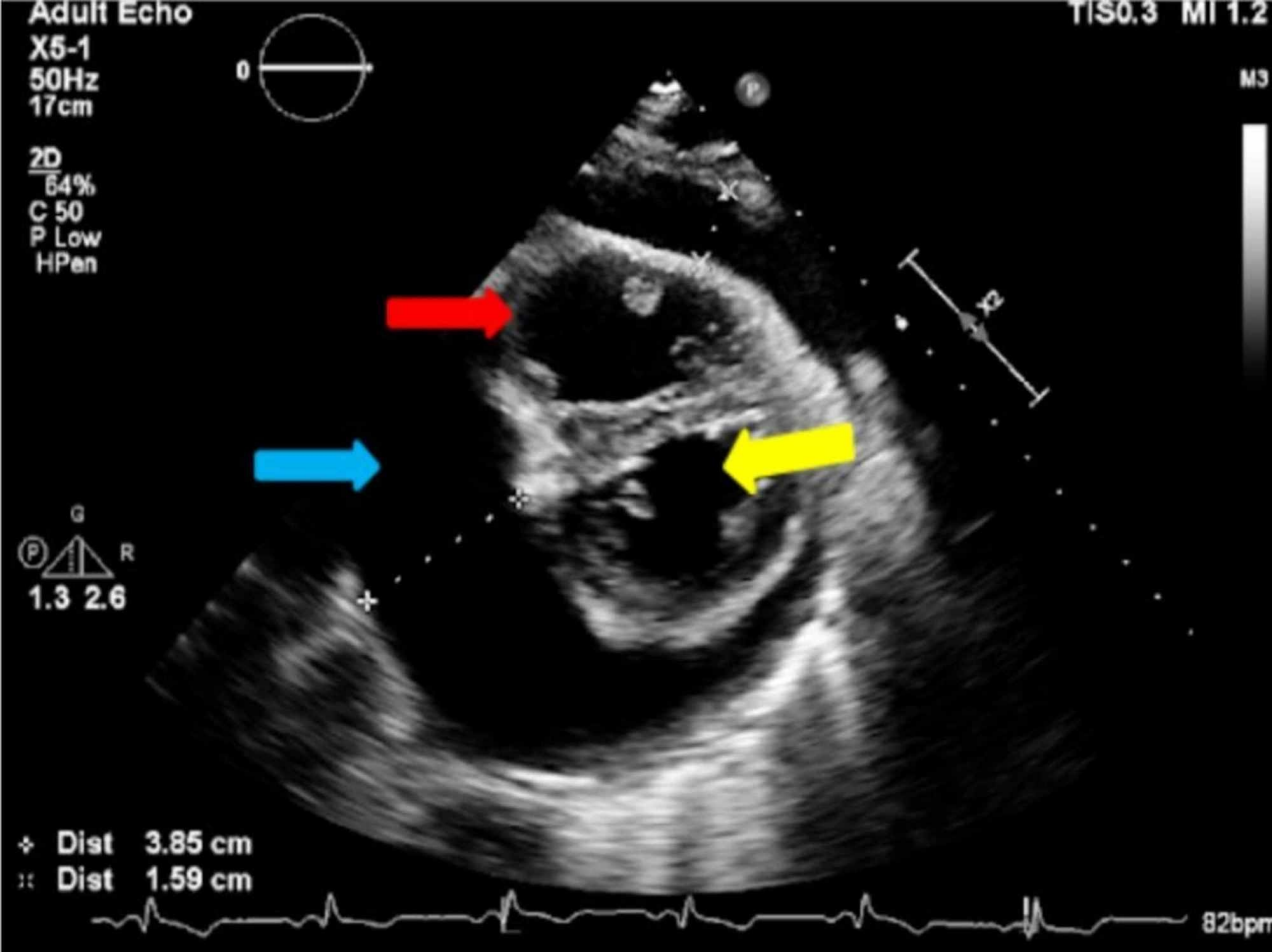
Parasternal short axis view of a large pericardial effusion measuring 3.85 cm (blue arrow), right ventricle (red arrow), and left ventricle (yellow arrow).
